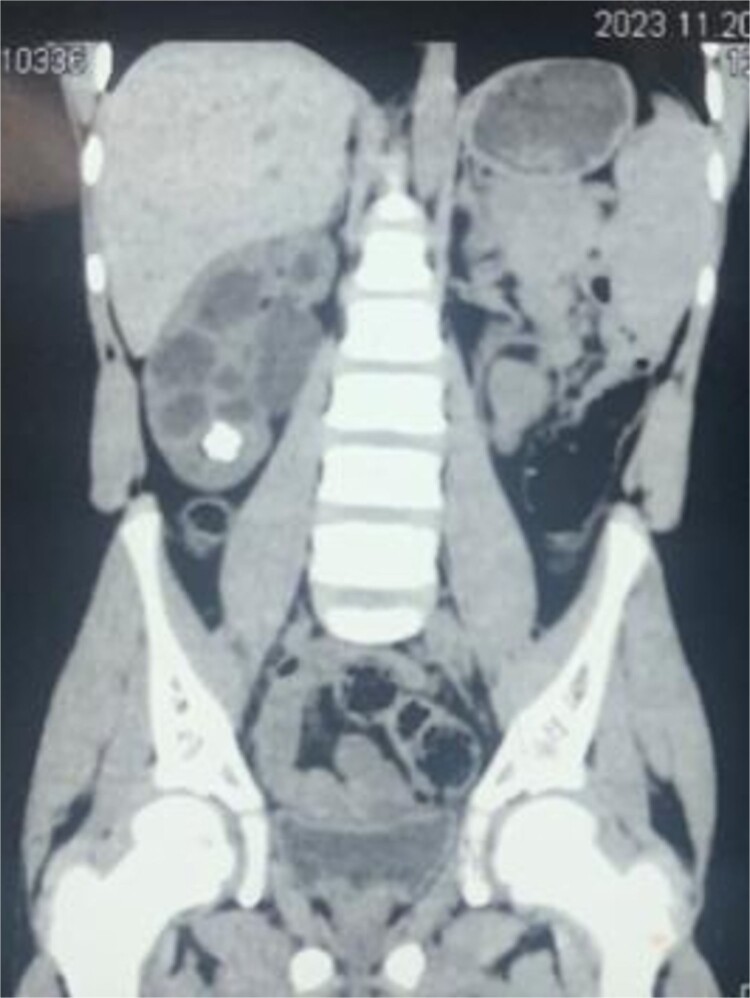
CT scan demonstrating stone in lower pole of right kidney.
